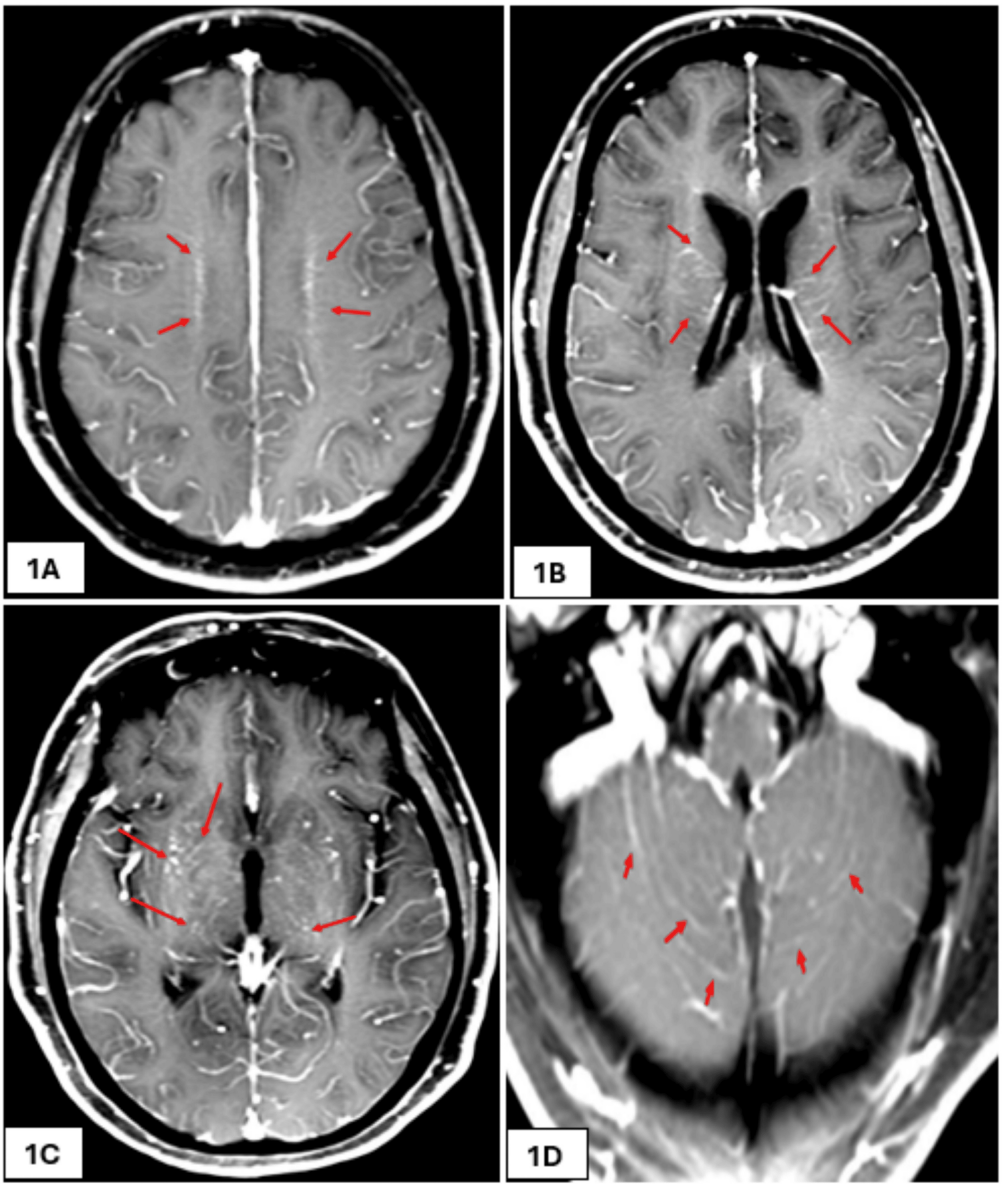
First MRI scan of brain (FAT-SAT axial images). Post-contrast T1 fat saturation (FAT-SAT) axial images showing bilateral symmetrical increased perivascular enhancement along the medullary veins - best appreciated at corona radiata level (long arrows in 1A) as well as basal ganglia and midbrain regions (long arrows in 1B and 1C) together with diffuse leptomeningeal enhancement extending to the scanned upper cervical cord (short arrows in 1D).
